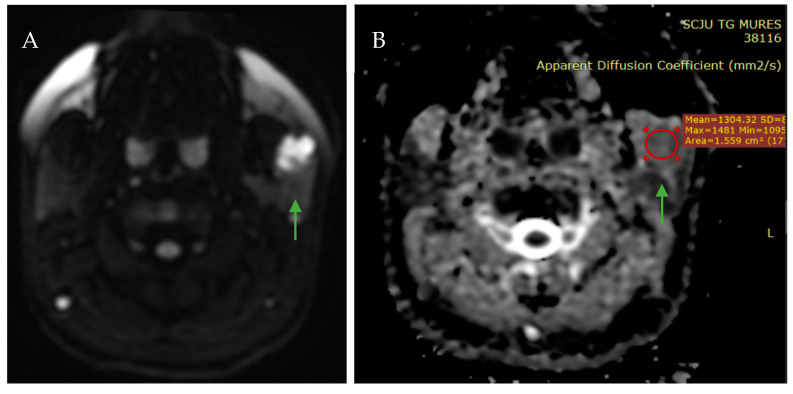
MRI axial sections showing the parotid gland mass (green arrows): (A) the axial DWI sequence demonstrating no diffusion restriction, and (B) the corresponding ADC map showing a high ADC value (1.3 × 10^−3^ mm^2^/s), the red circle indicates the area where the ADC value was calculated.
